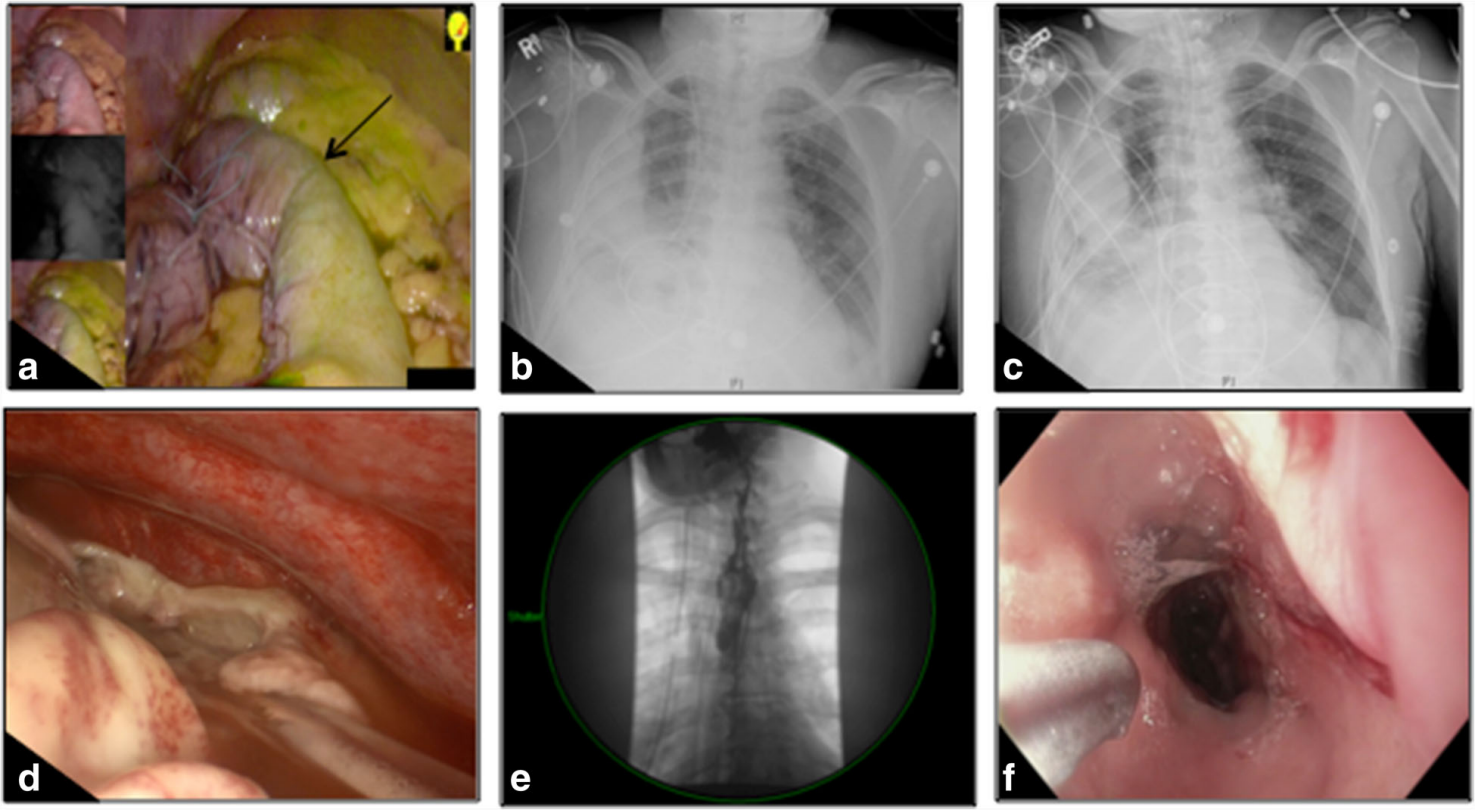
Case #1. A clear demarcation line between the well-perfused and marginally-perfused conduit was noted (*black arrow*, a). Postoperative chest X-ray demonstrated right-sided opacification despite an indwelling tube (b), which did not significantly improve with placement of an additional tube (c). On thoracoscopic exploration, a multiloculated effusion with significant rind was seen (d). A partially-covered self-expanding metal stent was placed (e), and the conduit healed after 2 serial stents (f)
